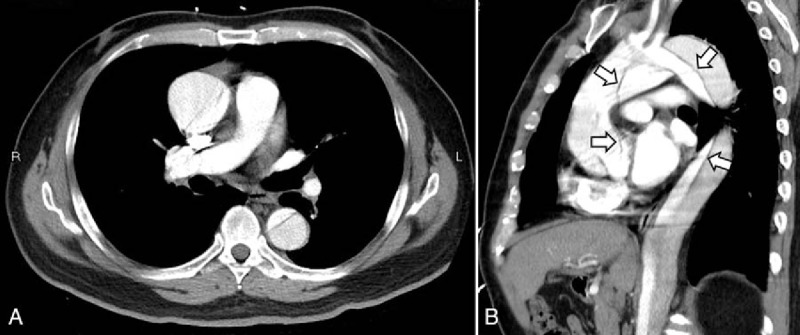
Contrast-enhanced computed tomography of chest indicated type A aortic dissection from aortic root. (A) Axial view; (B) sagittal view. Arrows indicate intimal flap.
